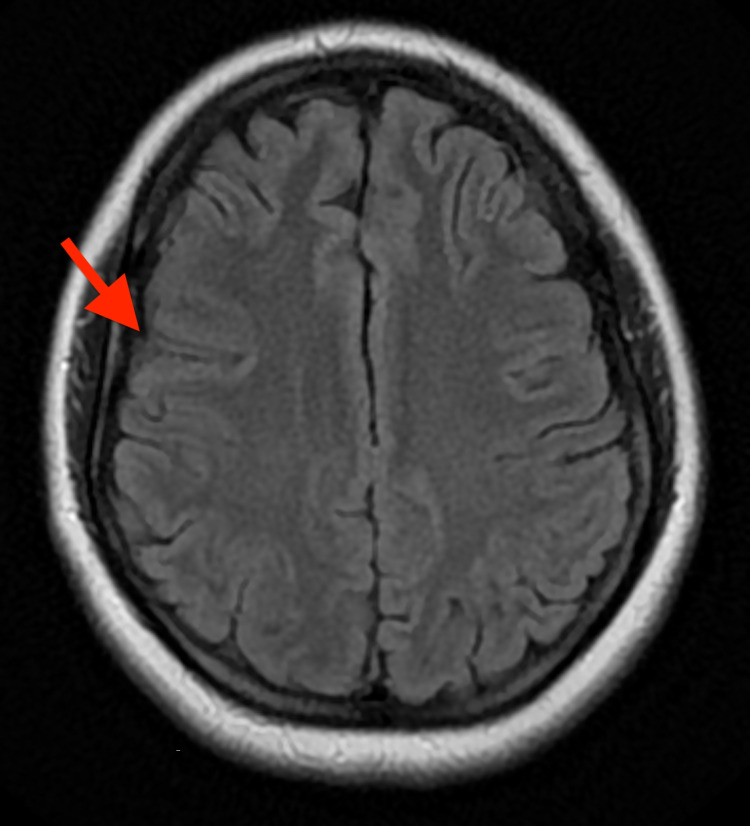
Axial view brain MRI showing a decrease in size of right frontal ring-enhancing lesion (red arrow).
